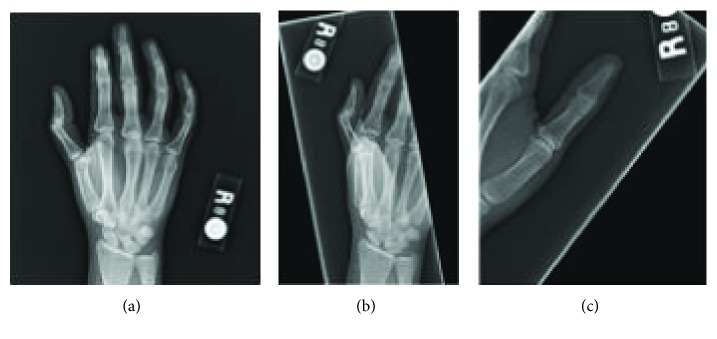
Injury radiographs. Obtained by urgent care on the day of injury. Radiology read stated no acute osseous abnormalities limited by patient positioning and pain due to decreased thumb range of motion. There is hyperextension present at the thumb MCP joint.
